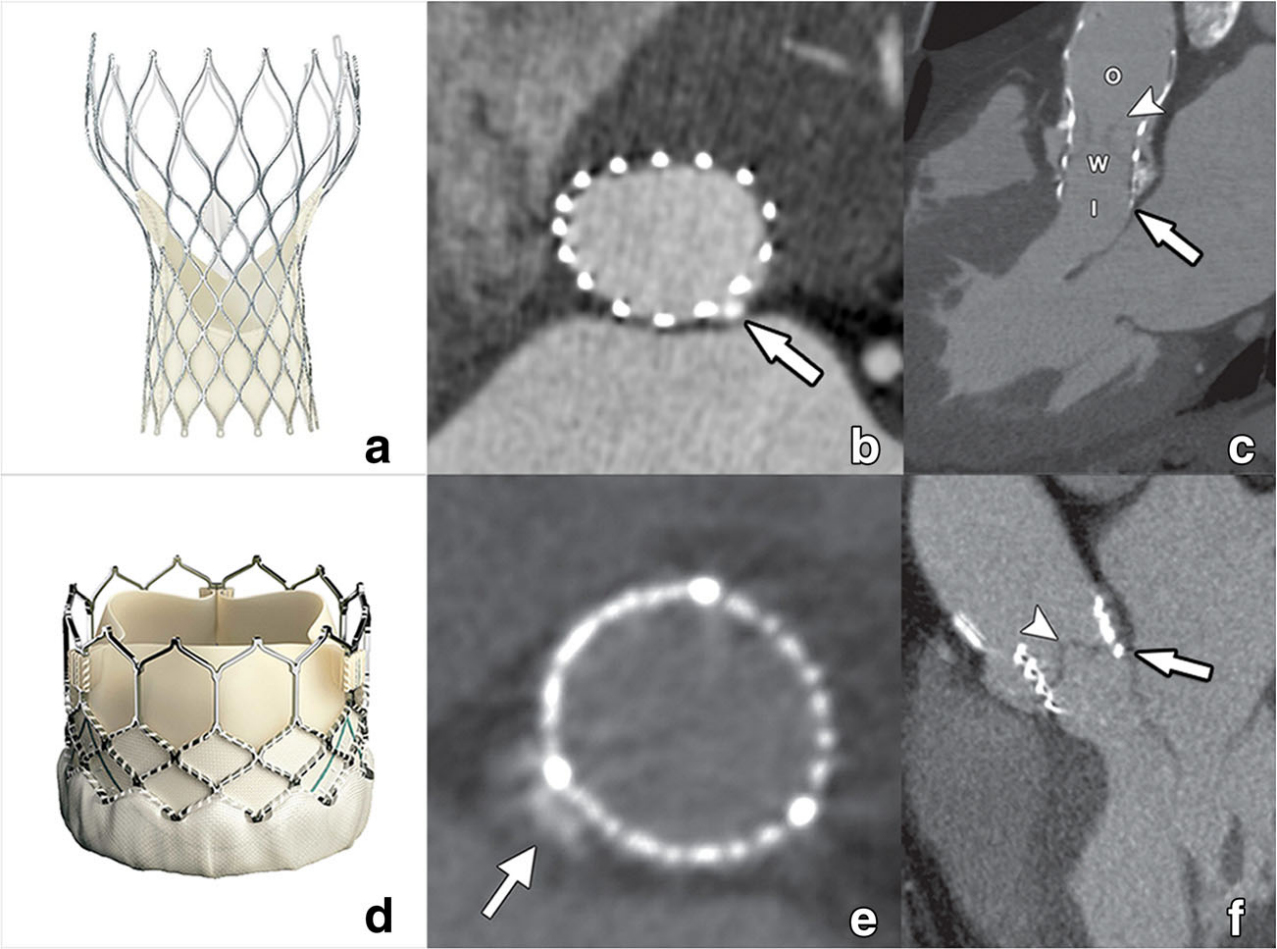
CT images of a Medtronic self-expandable Corevalve (a) and Edwards Lifesciences balloon-expandable Sapien valve (d). After deployment, a self-expandable valve will conform itself to the normal annular contour, acquiring an oval cross-sectional morphology (b). Compared with a balloon-expandable valve, the self-expandable Corevalve is larger in size and contains an inflow (I), waist (w), and outflow (O) functional part (c). This outflow part is by design intended to extend into the ascending aorta, covering but not obstructing the coronary ostia. The balloon-expandable Sapien valve (d) is shorter, with a mostly circular cross-sectional contour after deployment (e) as it forces this circular morphology on the annulus through the radial forces of the expanding balloon. In contrast to the self-expandable Corevalve, it remains within the aortic sinus (f). Within both THVs, the pericardial leaflets can be appreciated as fine hypodense linear structures (arrowheads in c, f), its visibility dependent on image quality. Small interposing calcifications (arrows in b, e) have in these examples only a minimal effect on THV expansion. Reused from reference [40], with permission
